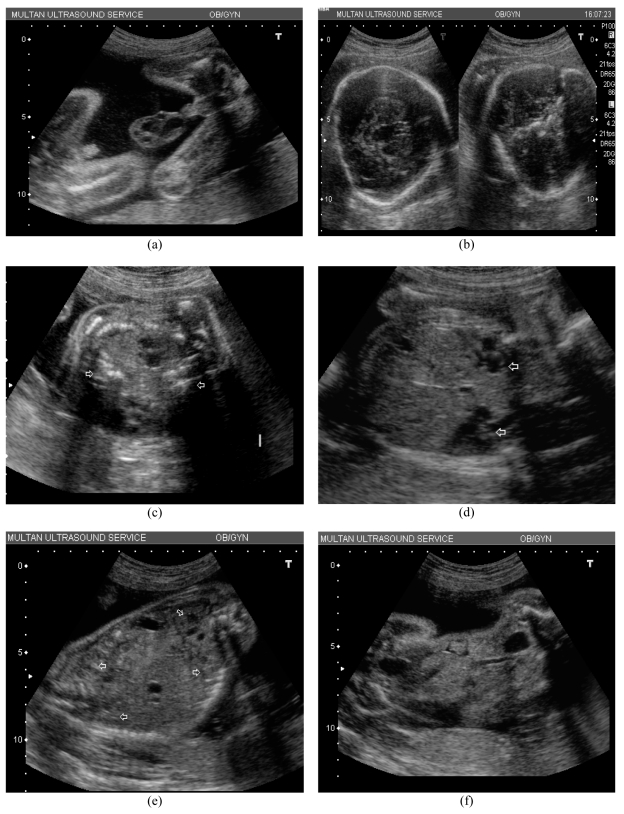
a) Transverse ultrasound sections of the fetal head; note delta shape (left) and absence of cerebellum and posterior fossa (right); b) transverse section of the fetal thorax, note two spines on opposite sides of a fuse thorax, arrows mark vertebrae; c) transverse section of the fetal thorax, showing two unfused hearts (arrows); d) transverse section of the upper abdomen, four kidneys are seen (arrows); e) an oblique transverse section of the fetal abdomen, note the two bladders, the way the fetal lower abdomens angulate and the obliquity of the section, this is a relatively coronal section and the fused liver and unfused bladder are seen in both fetuses; f) the two pelvises and external genitalia and four thighs are seen as the lower unfused abdomens diverge; a cross section of the cord shows multiple vessels.
